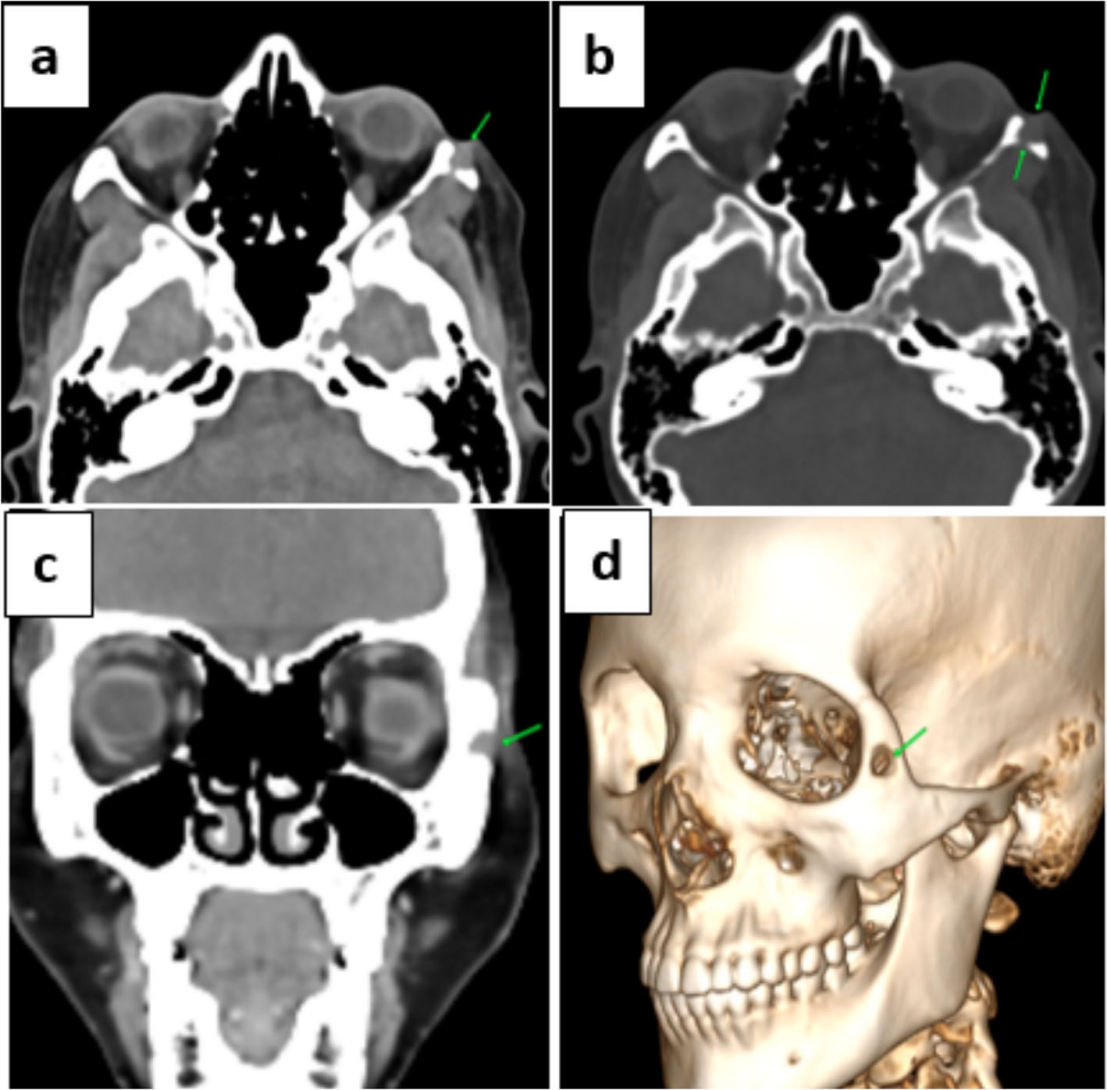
Left zygoma Osteomyelitis. a axial NCCT (soft tissue window), b axial NCCT (bone window), (c) coronal CECT (soft tissue window) & (d) oblique sagittal VRT images of the face show an ill-defined osteolytic lesion involving left zygomatic bone with associated sinus tract and mildly enhancing soft tissue thickening (green arrows). NCCT: Non-contrast Computed tomography, CECT: Contrast-enhanced Computed tomography, VRT: Volume Rendering Technique
